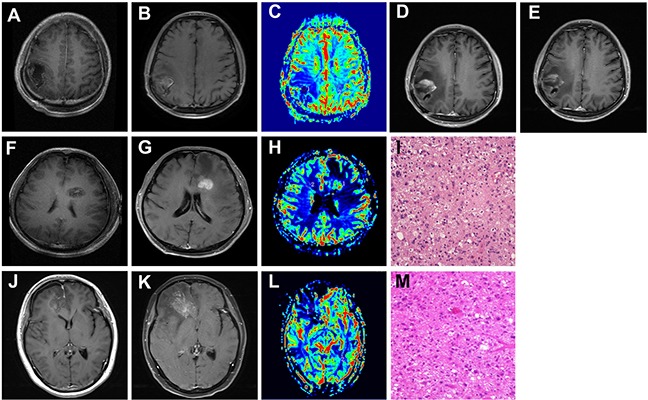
The clinical application of CTC in distinguishing tumor recurrence from radionecrosis A, F, G. Contrast axial T1-weighted image. After gross total resection, there is a surgical cavity without enhancement. B, G, K. Contrast axial T1-weighted image. After completion of RT, there is a new enhancing mass lesion on the initial post-RT MRI. C, H, L. rCBV map showed hypoperfusion (C and L, Patient 1 and Patient3) or hyperperfusion (H in patient 2) of the enhancing lesion (ROI 1) when compared to the contralateral normal white matter (ROI 2). D. and E. Follow-up of MRI performed 3(D) and 4(E) months after the initial post-RT MRI (Patient 1). I, M. Pathological findings in the second operation: tumor recurrence of GBM (Patient 2 and 3, HE, 10 x10). Patient 1: image A-E. Patient 2: image F-I. Patient 3: image J-M.
